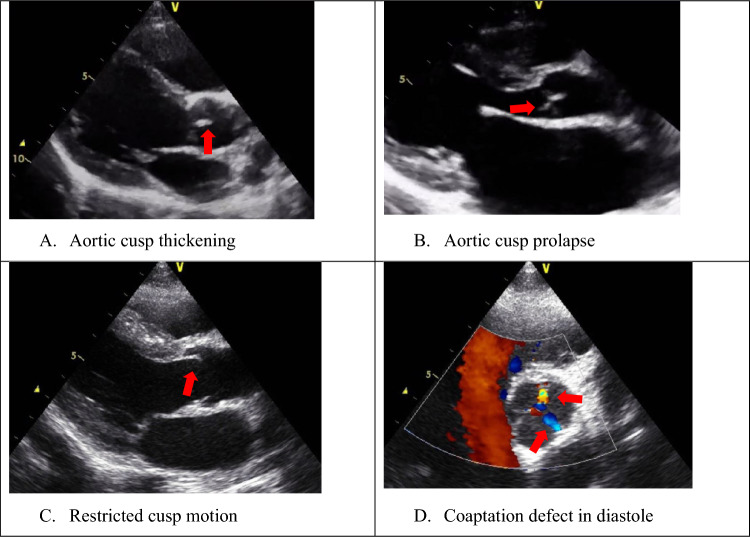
Aortic valve abnormalities associated with rheumatic heart disease. Parasternal long-axis views show A thickened aortic cusps (red arrows), B aortic cusp prolapse (red arrow), C restricted cusp motion (red arrow), and D parasternal short-axis view of the aortic valve in diastole demonstrating coaptation defect along the free edges of the valve with regurgitation seen in yellow and blue colour Doppler (red arrows)
